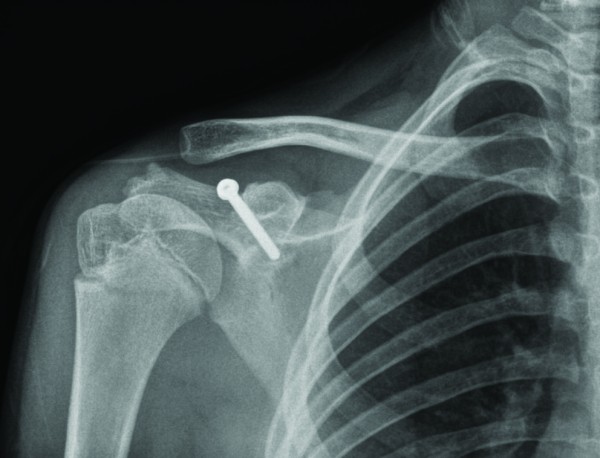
Post-operative radiograph after removal of threaded pins, with reduction of acromioclavicular joint maintained.
